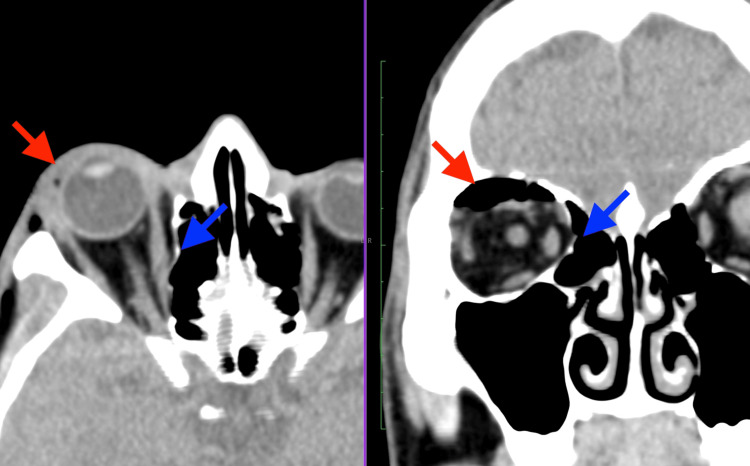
Axial and coronal view of CT soft tissue window show focal indentation of the right lamina papyracea (blue arrows) into the ethmoid sinus and right orbito-palpebral emphysema (red arrows) with air mass at the right superior orbit.
